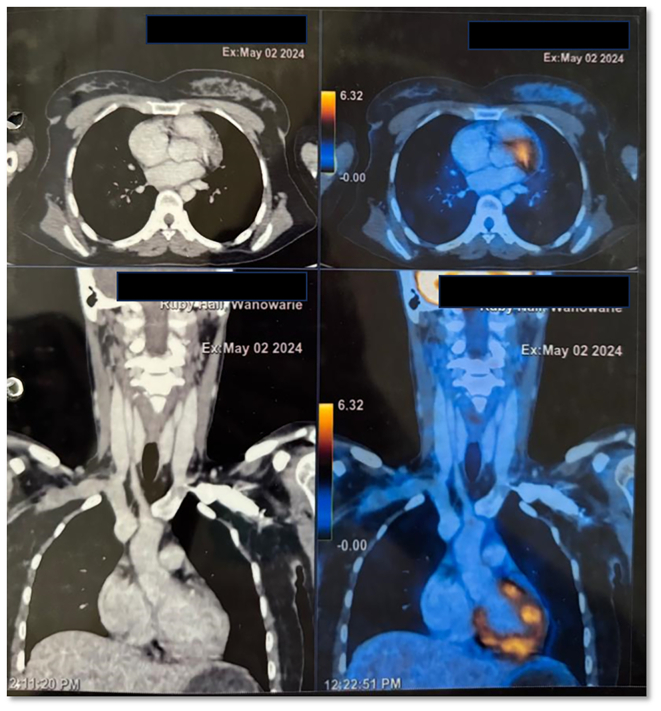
FDG uptake (SUVmax 3.2) noted in upper inner quadrant of left breast.
